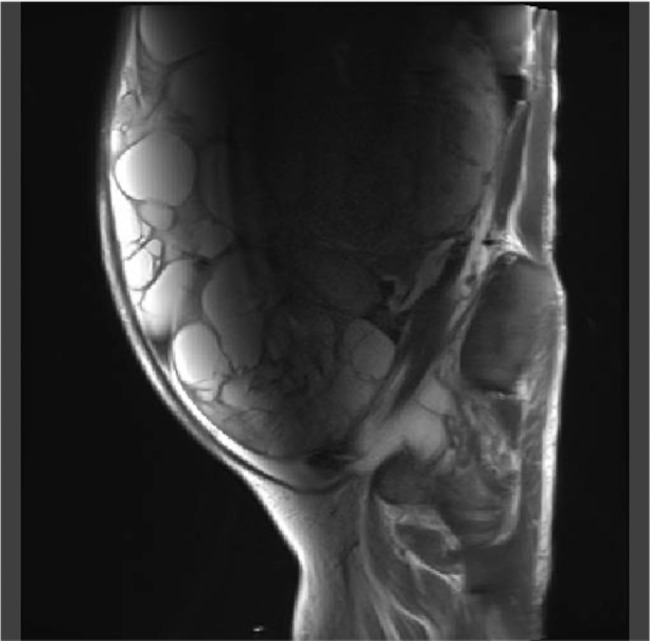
Magnetic resonance imaging (MRI) revealed an abdominal mass measuring up to 30 cm
